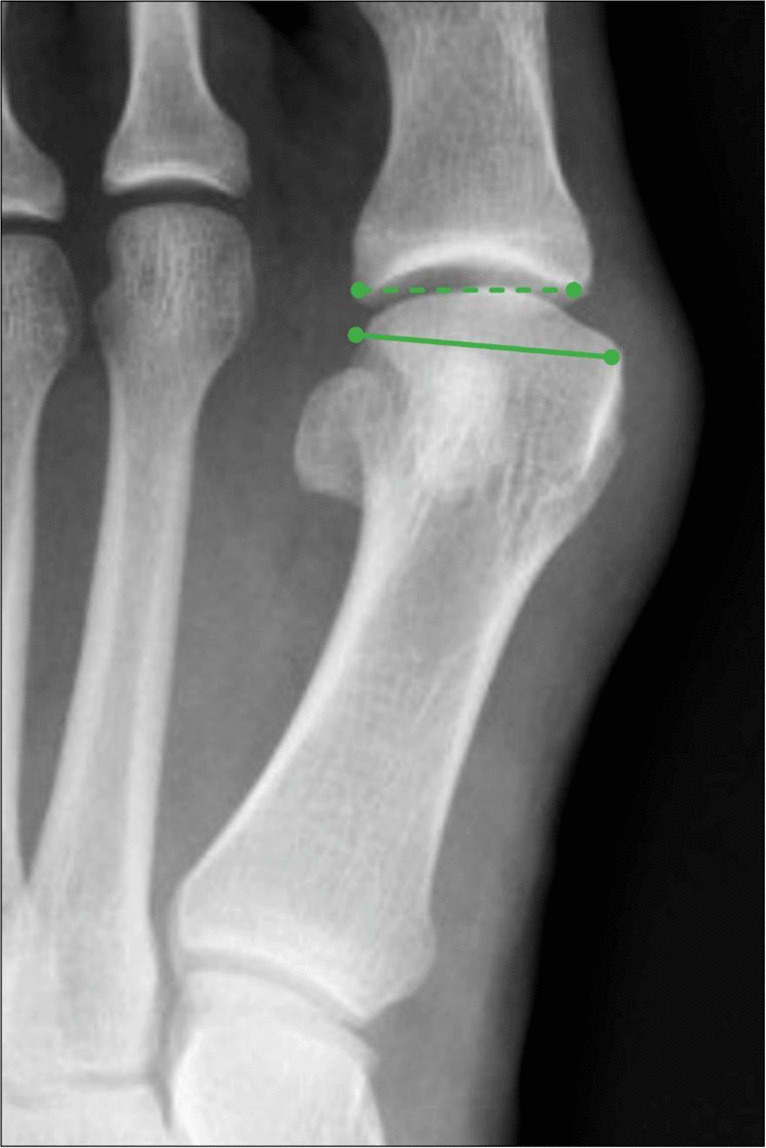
Measurement of the metatarsophalangeal joint angle on weightbearing DP radiograph of the foot. The angle is formed by a line (dashed line) connecting the medial and lateral margins of the proximal phalangeal base articular surface and a line (solid line) connecting the medial and lateral margins of the first metatarsal head articular surface. Normal angles are < 10° and indicate a congruent joint, and larger angles indicate greater metatarsophalangeal incongruency
